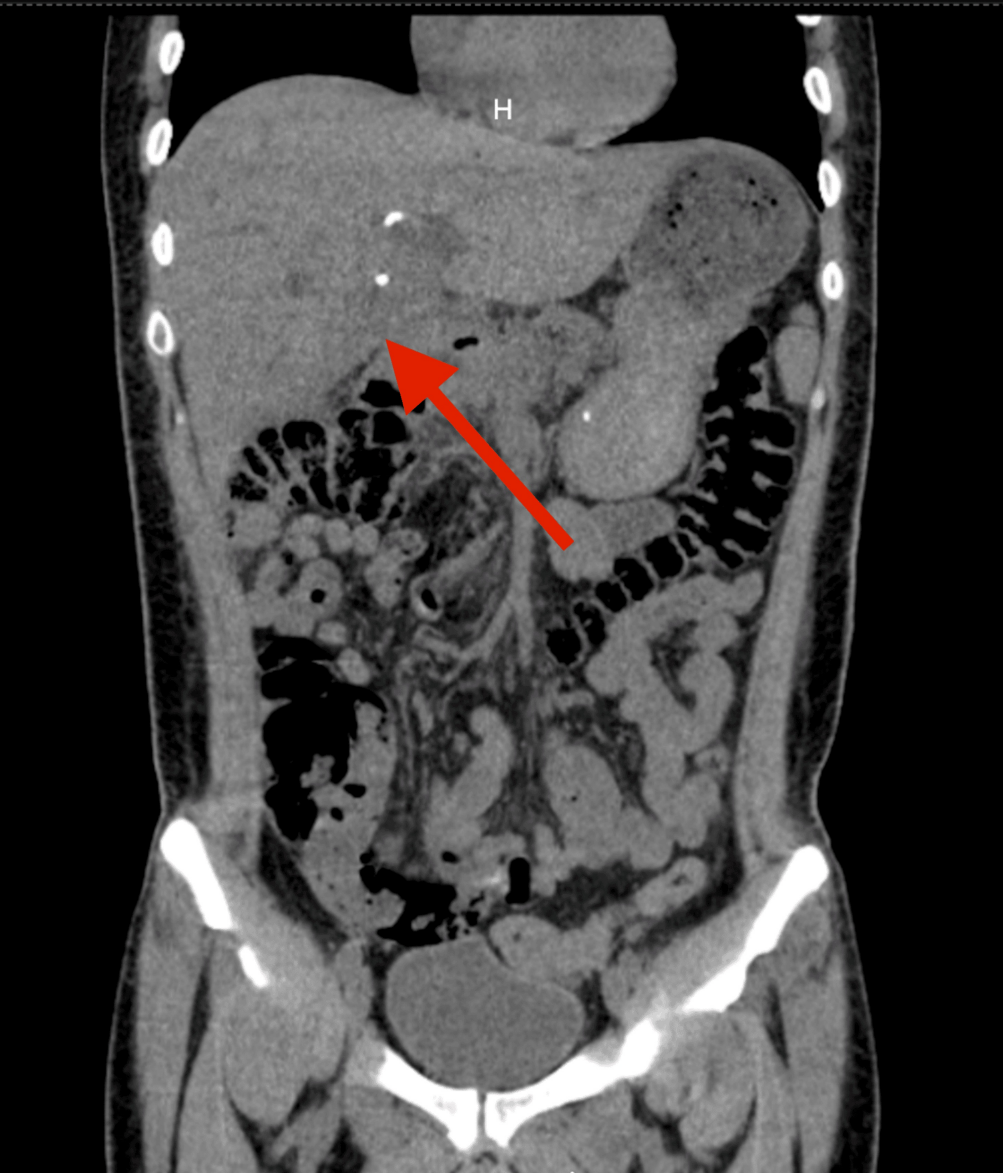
Abdominal and pelvic non-contrast computed tomography The comparative coronal images with the initial scan demonstrate an objective reduction of the tumor lesion, indicated by the red arrow.
